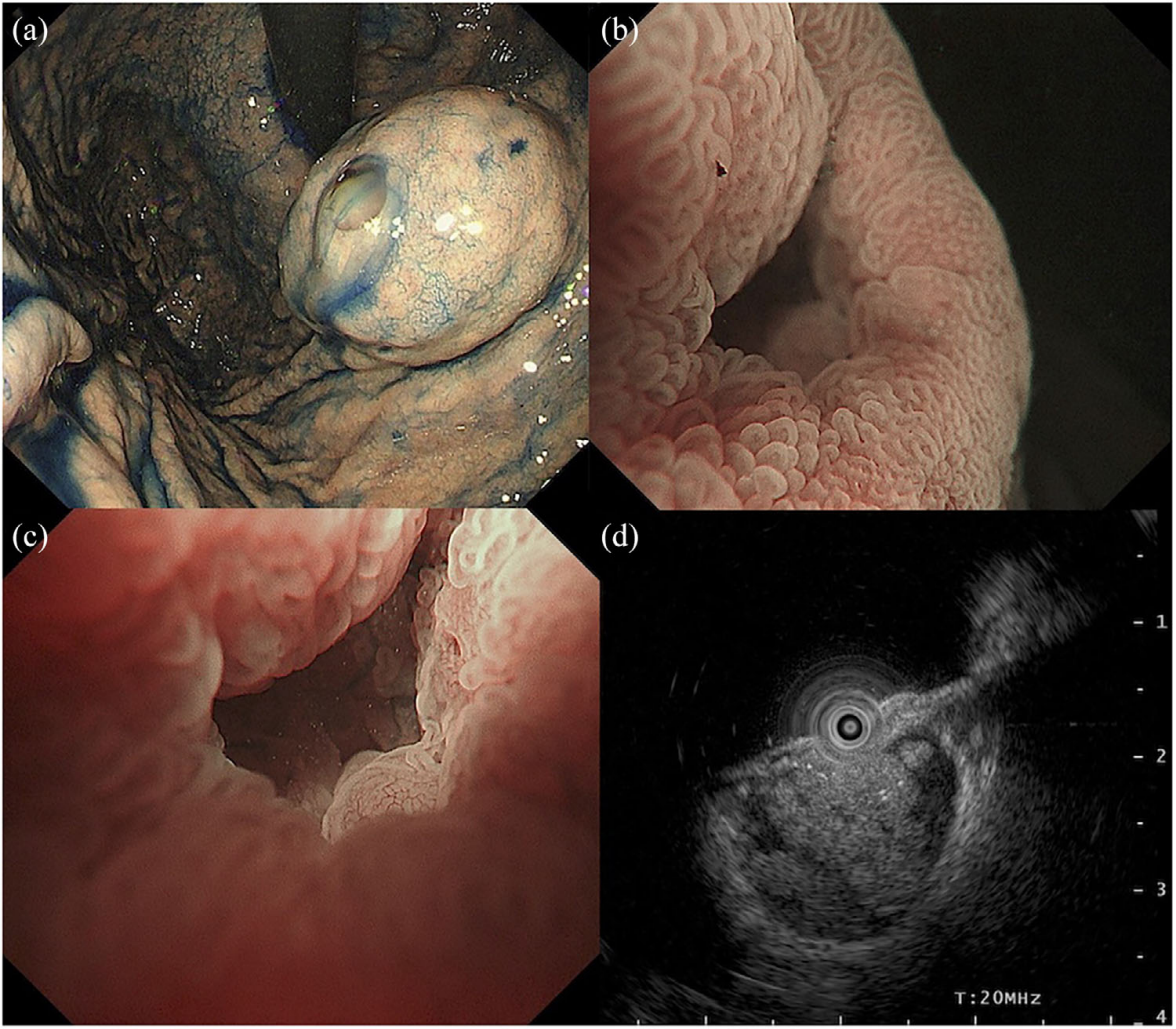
Detailed endoscopic images. (a) No obvious changes in the gross features of the lesion were observed. (b) Magnified endoscopy with narrow‐band imaging showed no evidence of intraepithelial neoplasm on the surface of the tumor. (c) Magnified endoscopy with narrow‐band imaging showed enlarged surface microstructures at the margins and villous structures in the center of the dimple. (d) Endoscopic ultrasonography with a miniature probe showed a lobulated solid component within the cystic lesion.
